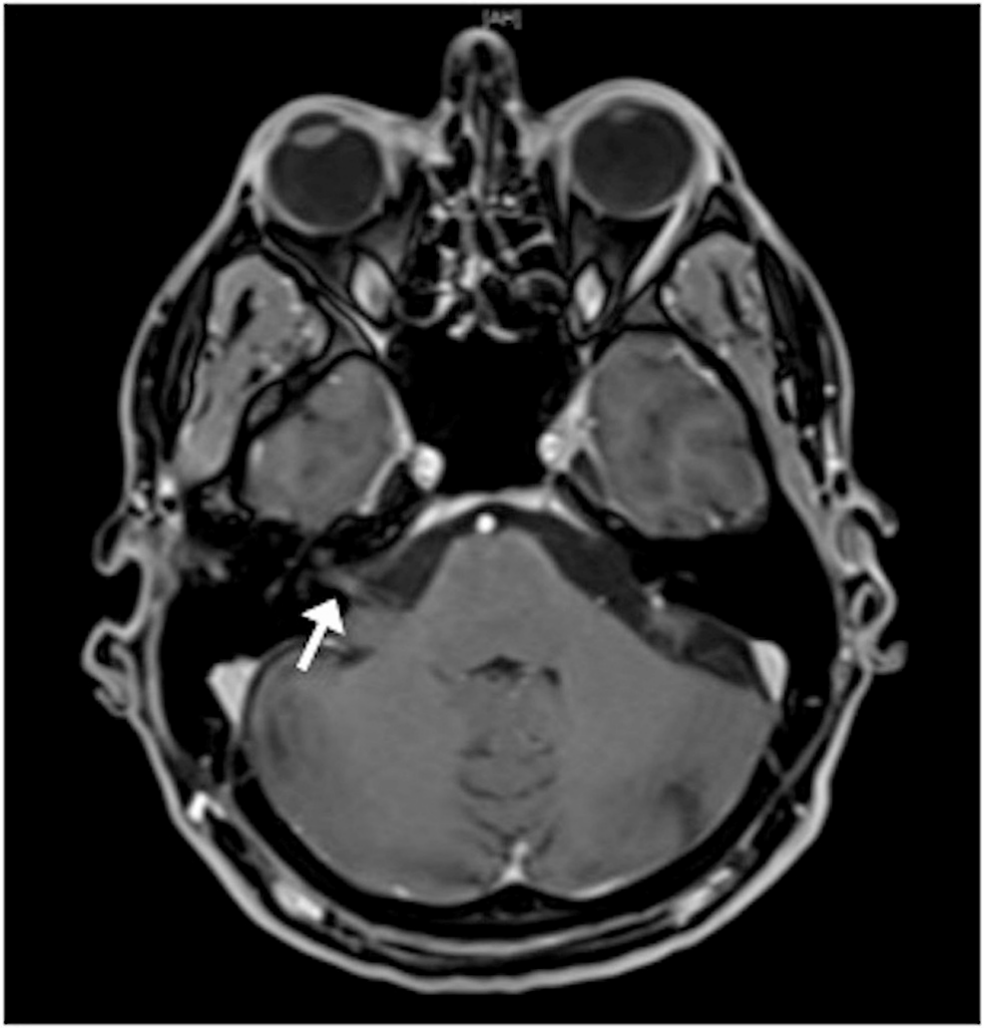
cMRI showing an enhancement of the right vestibulocochlear nerve. cMRI, cranial magnetic resonance imaging
